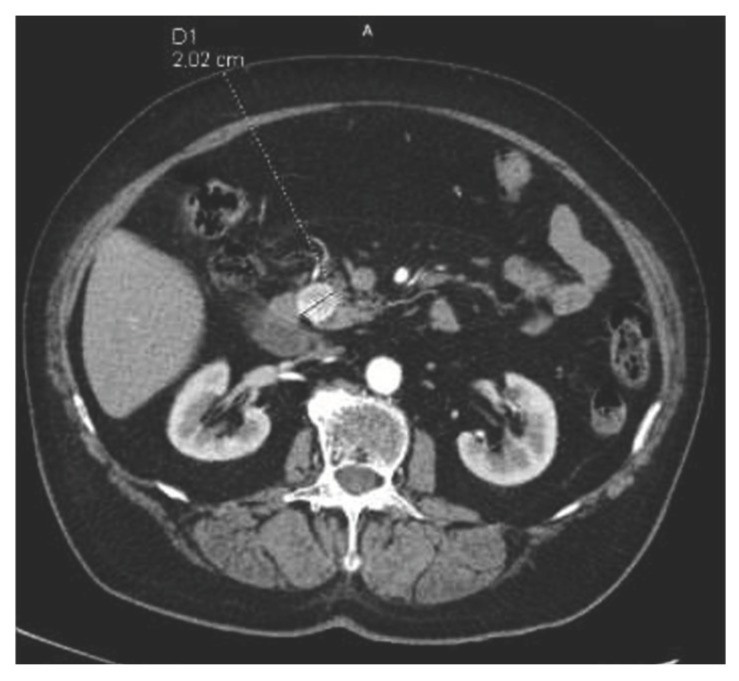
CT performed in June 2016: 20 mm tumor in pancreatic head.
